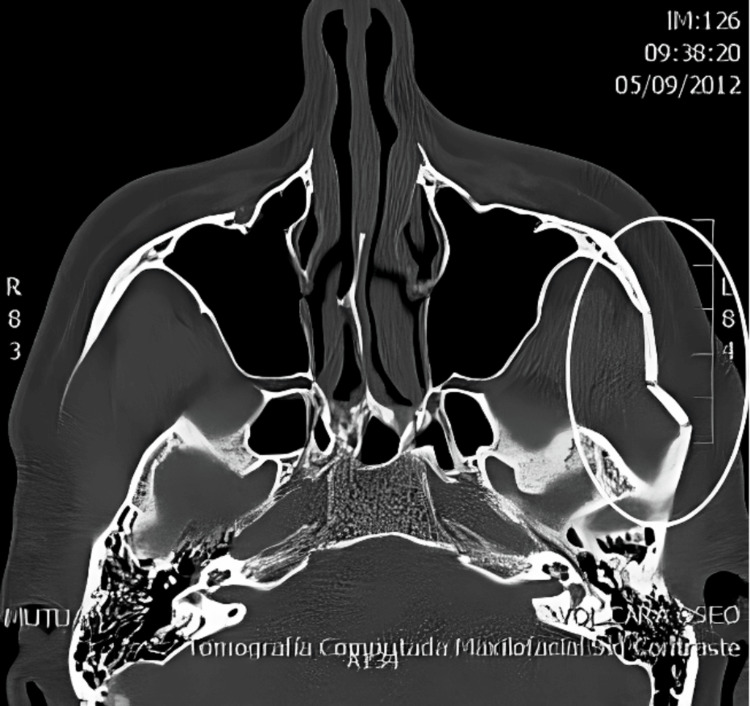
Preoperative non-contrast axial CT scan showing an “M”-shaped left zygomaticomaxillary complex fracture Reproduced from reference [16] (Creative Commons Attribution-NonCommercial-NoDerivatives 4.0 (CC BY-NC-ND 4.0)).
